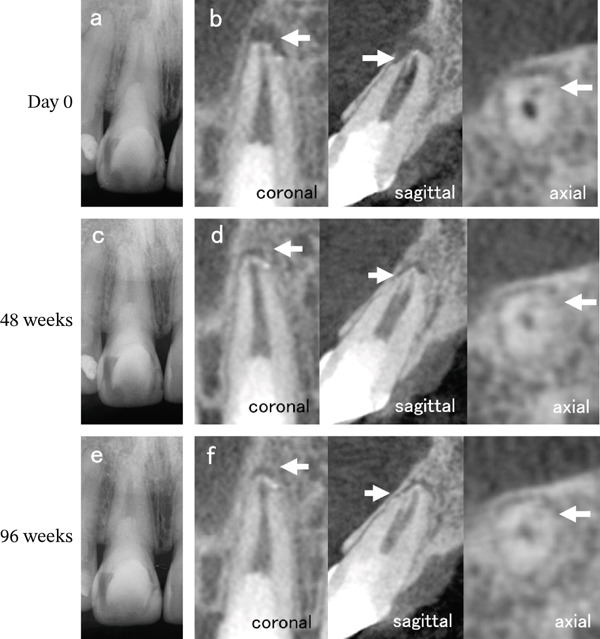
Case 2. Time‐course radiographic and CBCT evaluation of the maxillary right central incisor following DPSC transplantation. (a, c, e) Periapical radiographs and (b, d, f) CBCT images in coronal, sagittal, and axial views obtained immediately after transplantation (Day 0; a, b) and at (c, d) 48 and (e, f) 96 weeks postoperatively. Arrows indicate the perforation site near the root apex. Day 0: Open apical perforation and periapical radiolucency are visible. 48 weeks: increasing radiopacity within the root canal, near‐complete sealing of the apical perforation by hard tissue, and regression of the periapical lesion. 96 weeks: further consolidation of the mineralized tissue at the perforation site and continued resolution of the periapical lesion. Clinically relevant findings: selective hard‐tissue formation at the perforation site and progressive healing of the periapical lesion without the use of conventional perforation repair materials.
